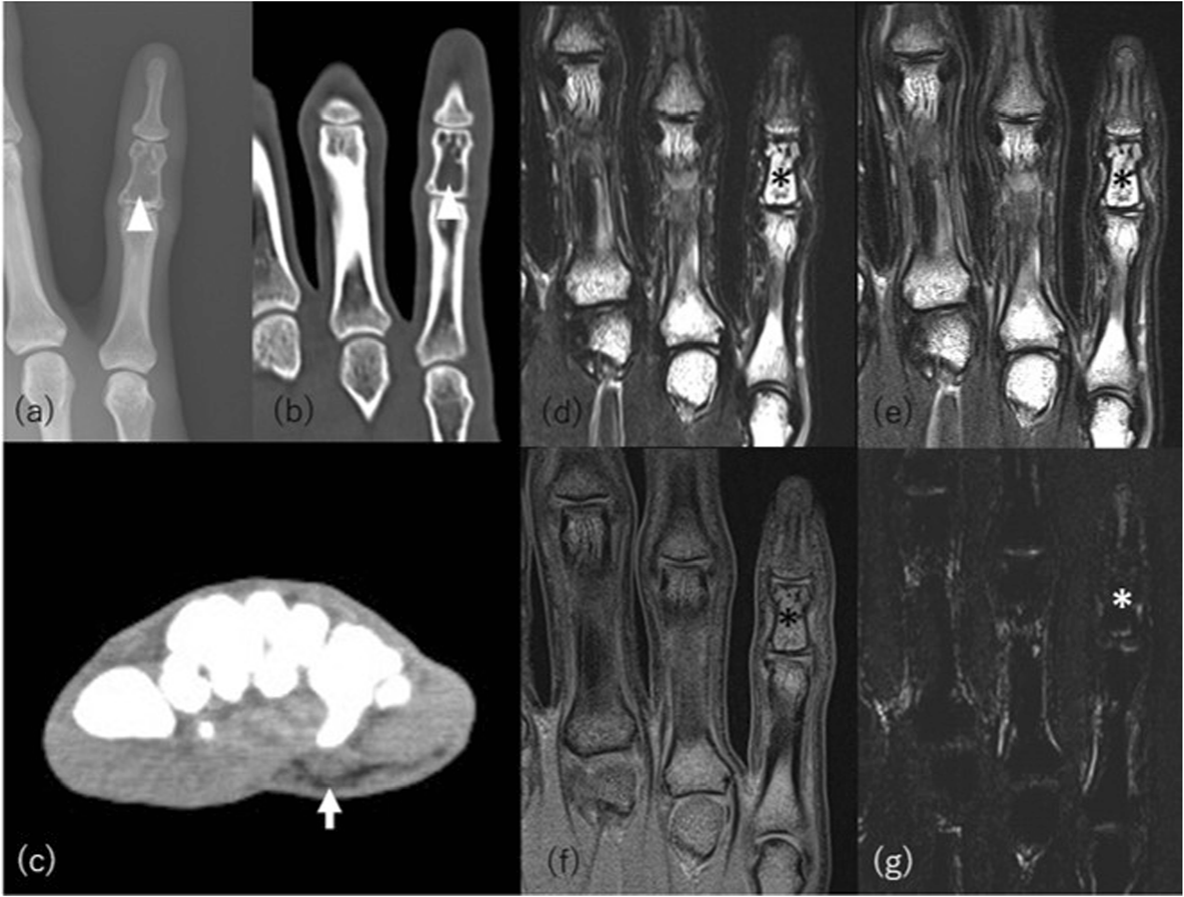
A 23-year-old woman with congenital generalized lipodystrophy and fifth finger pain. Lytic lesion (arrowheads) was evident on (a) radiograph and (b) CT in the fifth middle phalange. c CT showed almost complete loss of fat tissue other than the palmar fat pad (arrow). MRI showed complete lack of bone marrow adipose tissue. Coronal (d) T2-weighted and (e) STIR images show almost the same signal. f T1-weighted image shows no adipose high signal intensity. An asterisk denotes the referred lytic lesion. g DIXON-based fat image shows almost complete loss of fat high signal in the bone marrow and soft tissue
